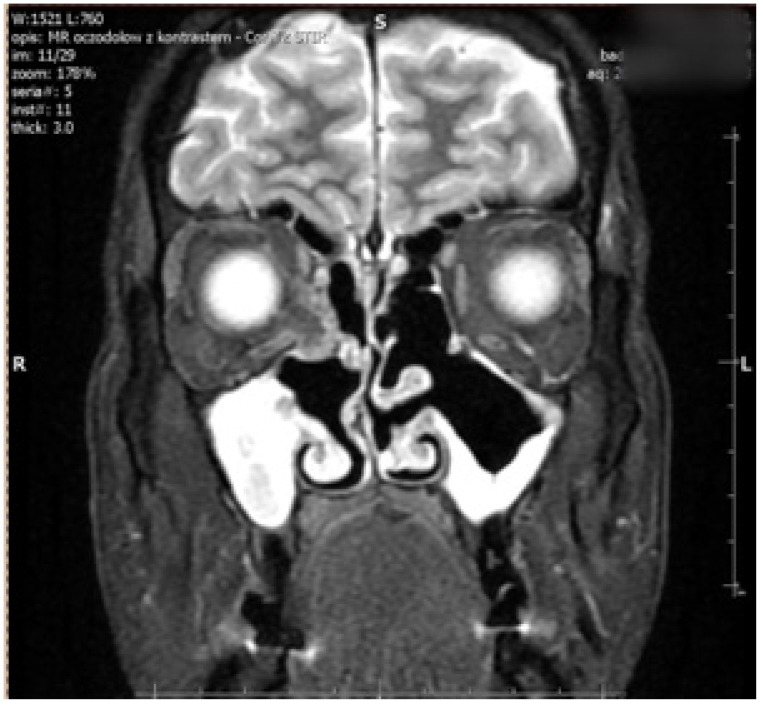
Postoperative coronal MRI scan showing bilateral ethmoidectomy, antrostomy, and uncinectomy in chronic maxillary sinusitis with significant mucosal thickening. MRI scan demonstrates the right medial rectus displacement.
